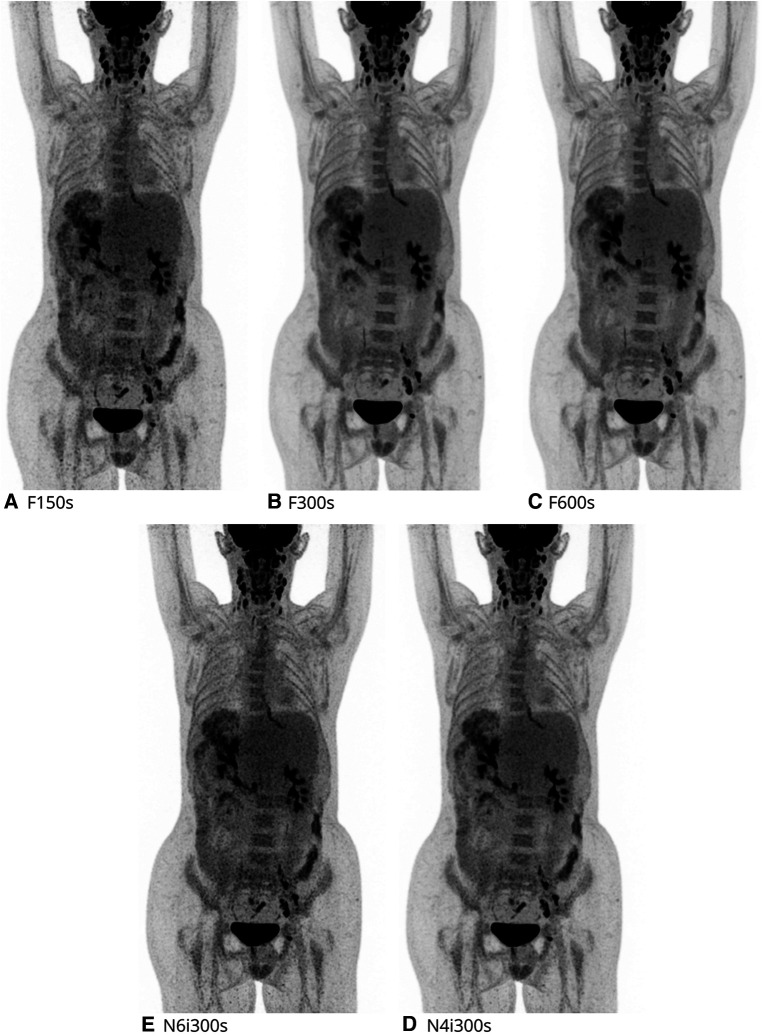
2-[18F]FDG maximal intensity projection (MIP) of each reconstruction evaluated. Here a 16y male, referred for recurrence of post-transplant-lymphoproliferative-disease.
